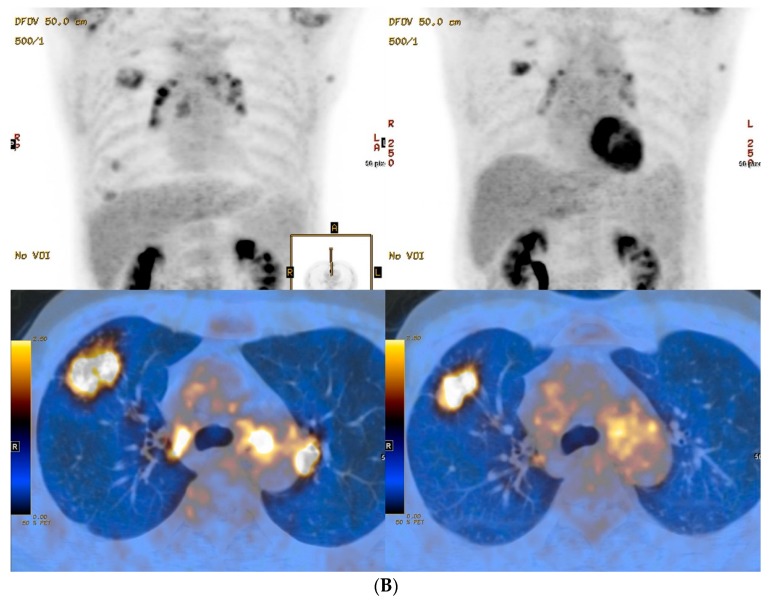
PET/CT images of lungs from two MDR-TB patients. Baseline (left column) and 12 months follow-up (right column) maximum-intensity projection and transverse fusion PET/CT images of patient 2 (A) and patient 4 (B). (A) Images of patient 2 show multiple hypermetabolic lesions in the right lung at baseline that disappeared in follow-up images despite persistent disease. (B) Images of patient 4 show multiple hypermetabolic lesions in the right lung at baseline that improved but were persistent in follow-up images irrespective of negative culture conversion and treatment success. Total metabolic lung volume (92.3 cm^3^) and total lung glycolysis (307.3 cm^3^) in patient 2 using a baseline PET/CT were much higher than for patient 4 (4.6 cm^3^ and 14.1 cm^3^, respectively).
